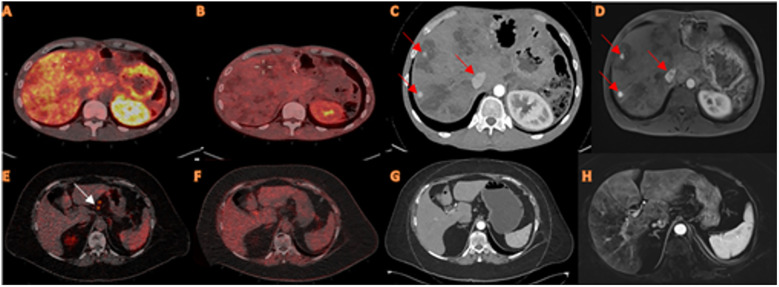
A 39-year-old man (DK-526) with MEN1 complicated by metastatic insulinoma to the liver. (A, B) 68Ga-DOTATATE PET/CT (A) and 18F-FDOPA PET/CT (B) did not identify uptake in the area of the focal enhancing lesions on CT/MRI. (C, D) CT (C) and MRI (D) show diffuse liver involvement with lesions ranging from 0.3 to 0.9 cm (red arrow). (E–H) Functional and anatomic abdominal imaging in a female with MEN1 (DK-143). (E) 68Ga-DOTATATE PET/CT demonstrates uptake of two metastatic lymph nodes near the gastric wall (white arrow), not seen on any other imaging (SUV maximum 35.5 and 31.5); white arrow. (F–H) 18F-FDOPA PET/CT (F), computed tomography (CT) (G), and magnetic resonance imaging (MRI) (H) failed to demonstrate the corresponding lymph nodes.
